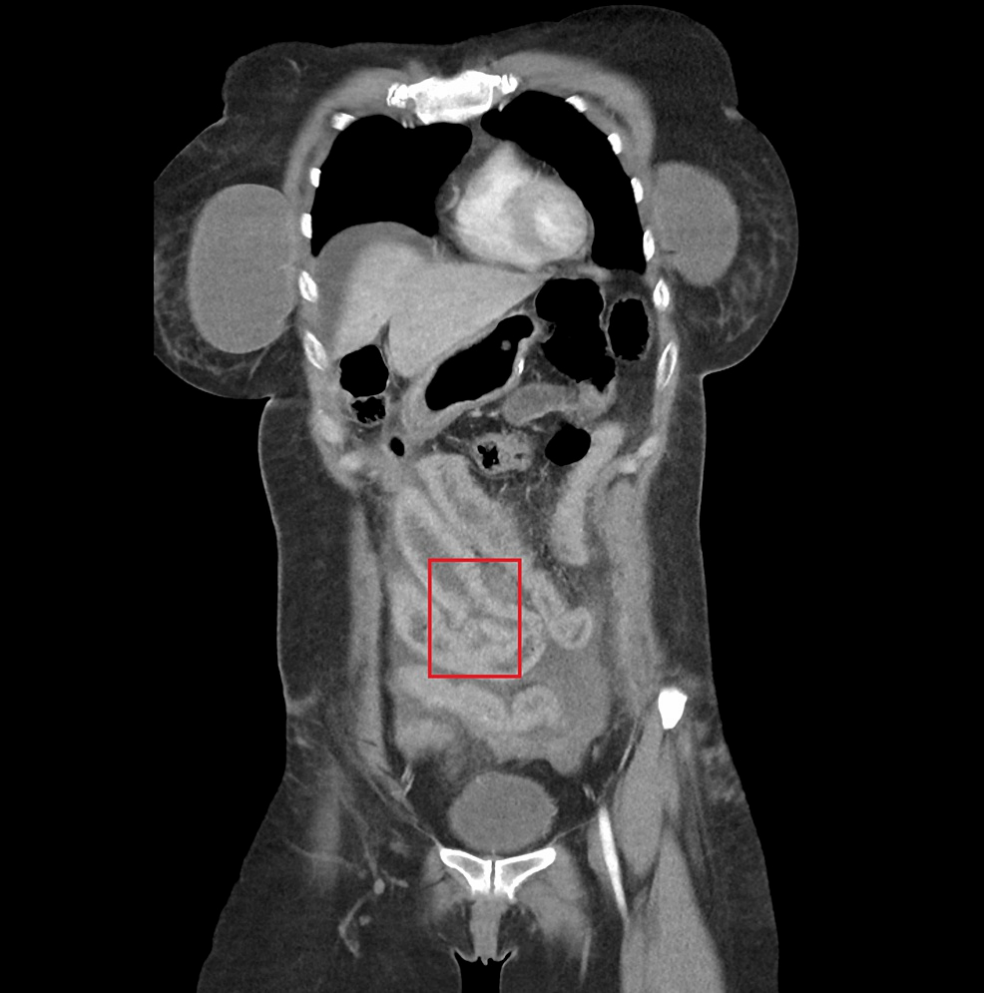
CT abdomen and pelvis showing SADI-S efferent loop wall disruption CT: computed tomography, SADI-S: single anastomosis duodenal-ileal bypass with sleeve
